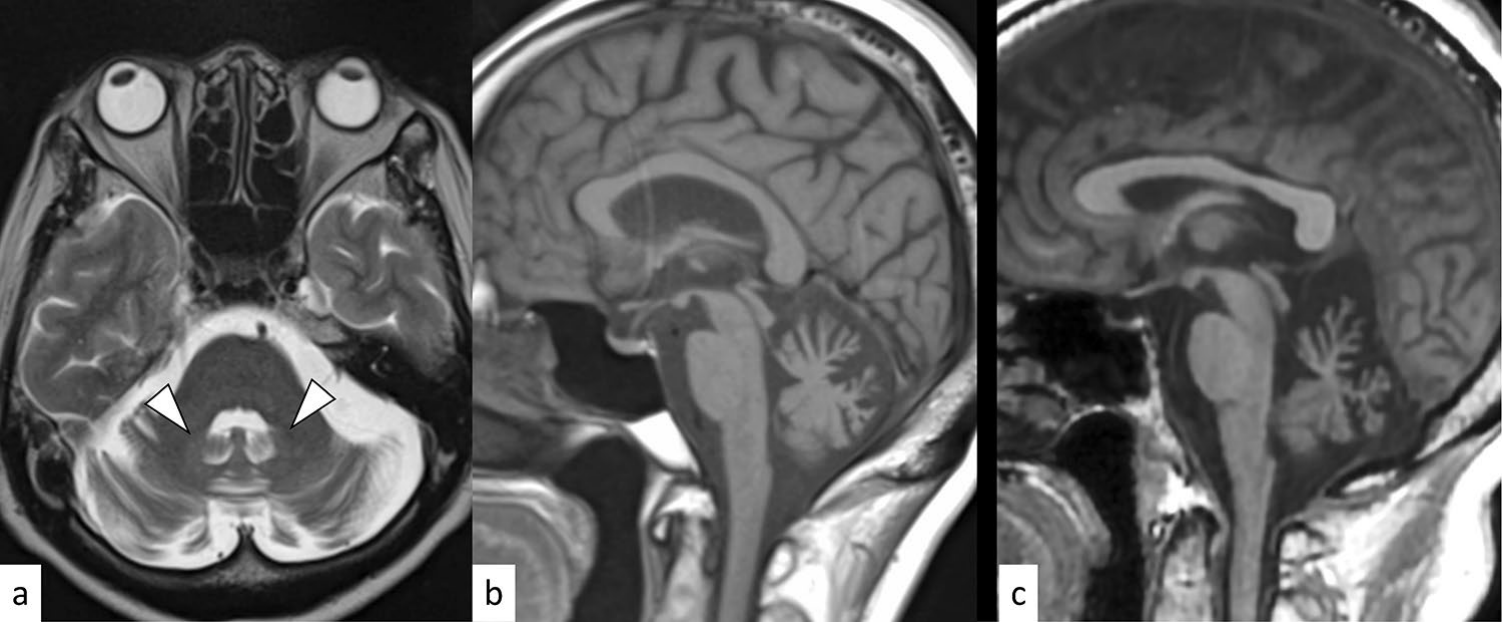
A 64-year-old woman with SCA6 (a, b) and a 50-year-old woman with familial hemiplegic migraine type 1 (c). T2-weighted axial image shows the absence of T2 hypointensity in the bilateral dentate nuclei (a, white arrowheads). The hot cross bun sign is absent (a). Pure cerebellar atrophy is observed on T1-weighted sagittal images in both patients (b, c)
